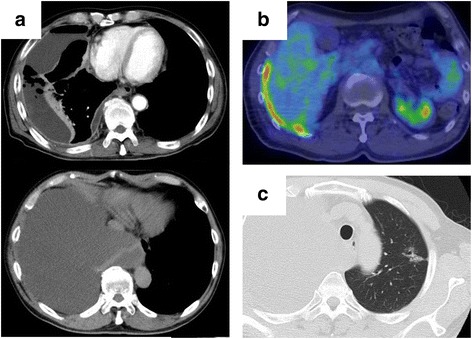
a Chest computed tomography (CT) at presentation showing *right* pleural effusion and focal thickening of the parietal pleura. b Positron emission tomography (PET) showing a moderate uptake of flouro-deoxy-glucose (FDG) with the maximum standardized uptake value of 6.6 in the thickened pleura. c Chest CT revealing a 2.5-cm “part-solid” ground-glass opacity (GGN) in the upper division of the *left* lung
